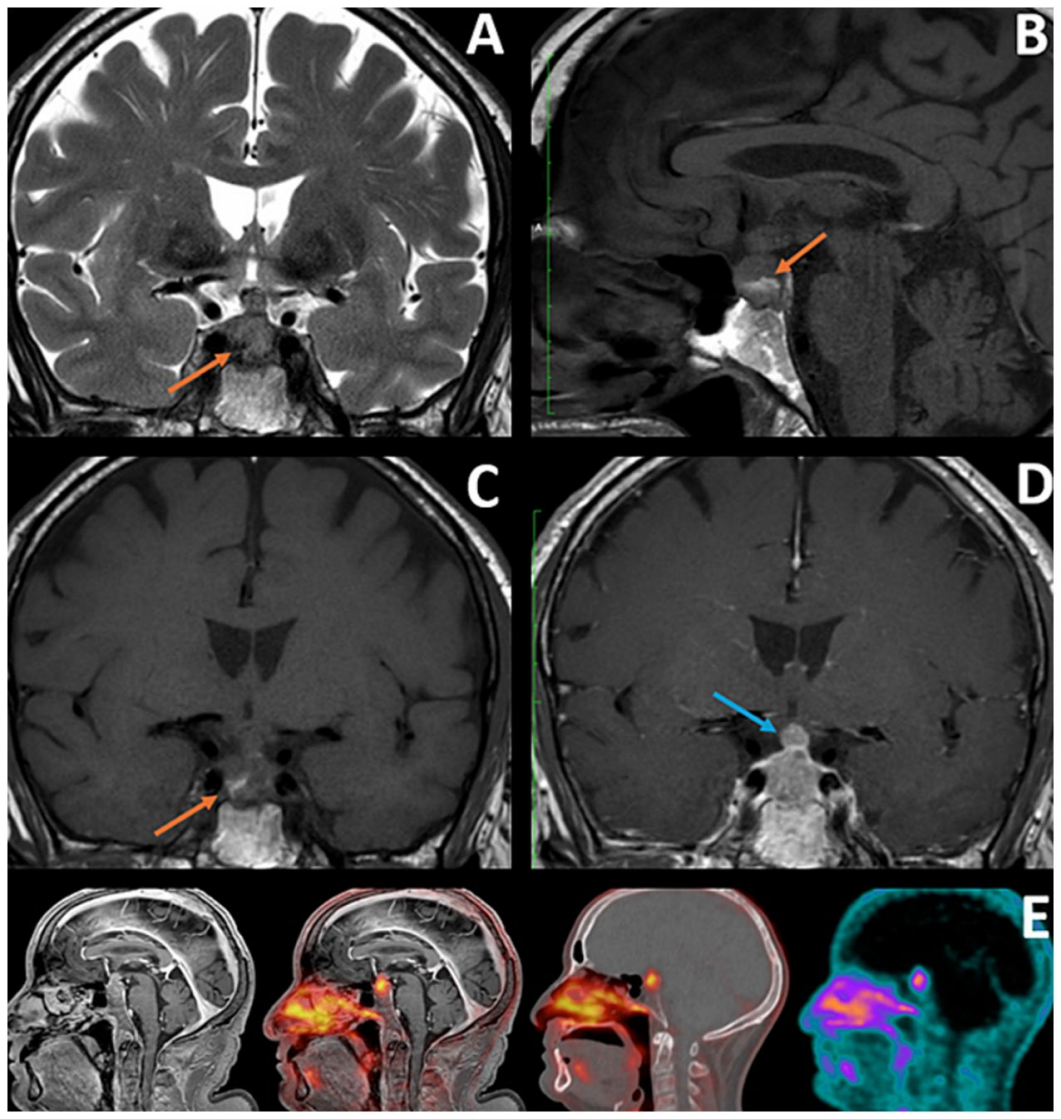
(A–C) Coronal T2, Sagittal, and Coronal T1: Orange arrows showing hemorrhagic component within the lesion, with hypointense signal in T2 WI and hyperintense signal in T1 WI (consistent with subacute character), which could be consistent with recent apoplexy of pre-existing pituitary adenoma (factor contributing to the initial misdiagnosis). (D) Coronal T1 with Gd: Blue arrow showing a nodular component of the lesion at the level of the pituitary stalk, a feature that, according to some researchers, may facilitate correct diagnosis of pituitary metastases. (E) IRM T1 TFE Gd; Fusion image PET/MRI; fusion image PET/CT; PET.
